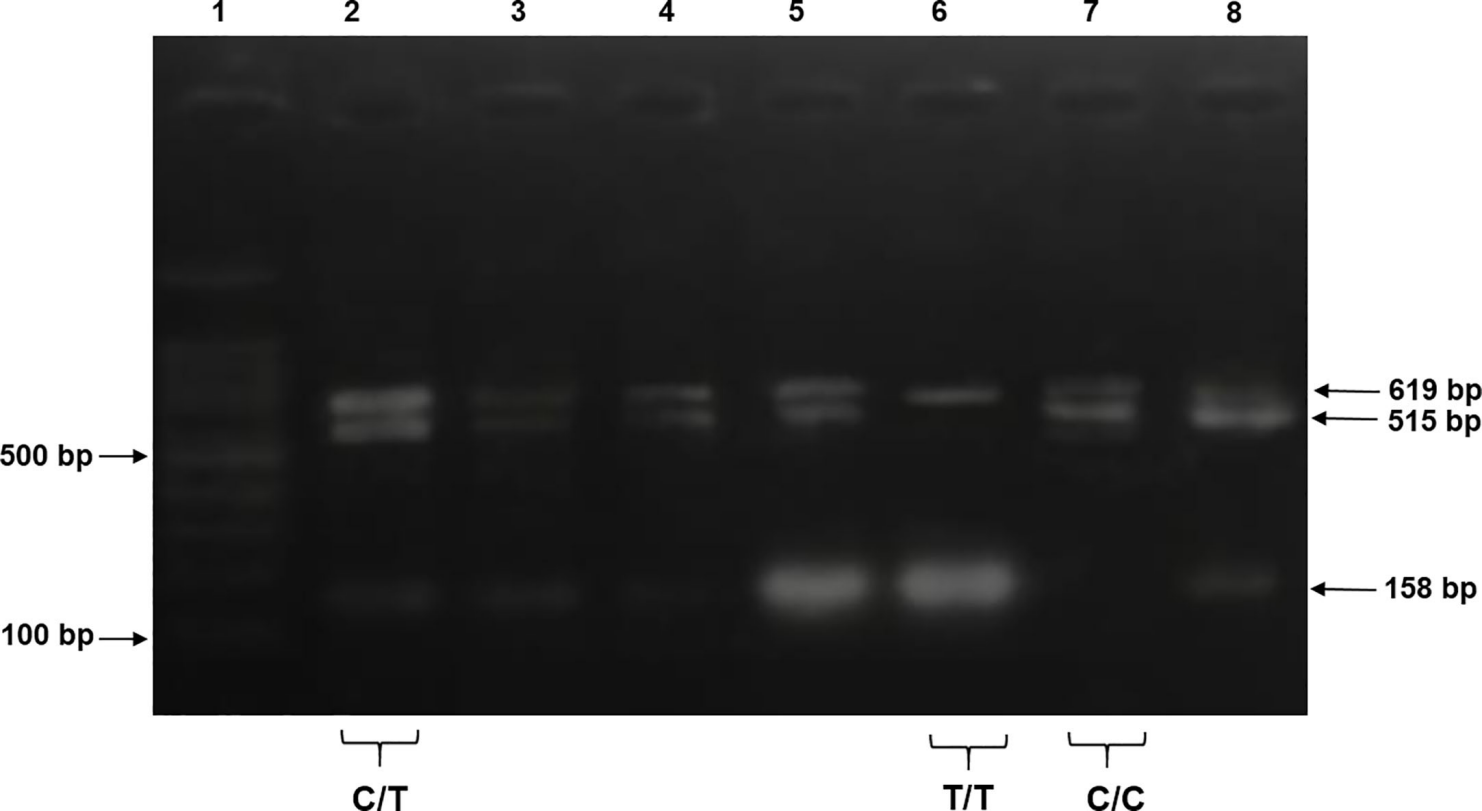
The products were electrophoresed on 2% agarose and directly visualized with ethidium bromide under UV light. Lane 1: 100 bp DNA ladder, lanes 2–5 and 8 indicate samples with heterozygous genotype CT CC (619 + 515 + 158 bp), lane 6 indicates homozygous genotype TT (619 + 158 bp), and lane 7 indicates a sample of homozygous genotype CC (619 + 515 bp).
